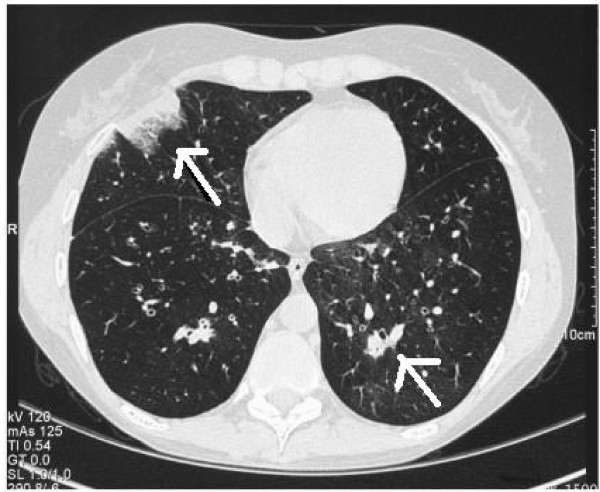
September 2005 (CT Thorax): Multifocal areas of infection.
